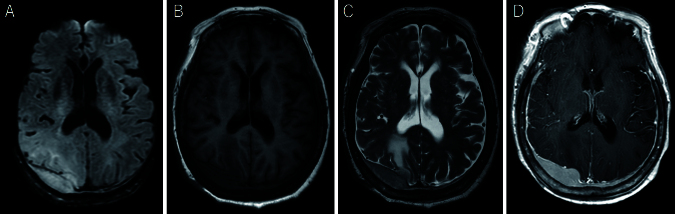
MRI. Dural-based extra-axial mass lesion showed slight hyperintensity in diffusion-weighted image (A), isointensity in T1-weighted image (B), and slight hyperintensity in T2-weighted image (C). Post-contrast scans showed homogeneous enhancement of the mass with a long dural tail sign (D). MRI: magnetic resonance imaging.
